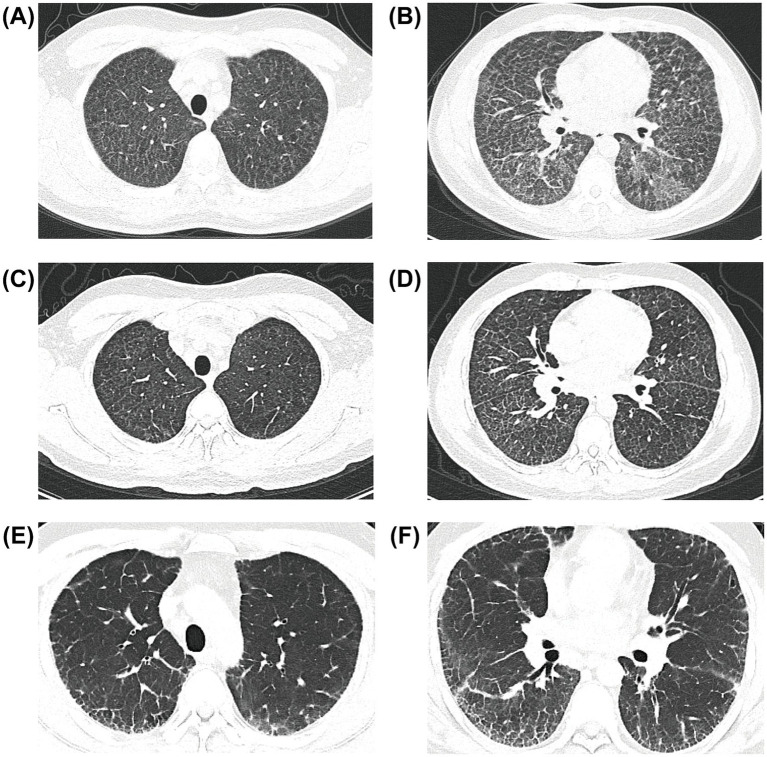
High-resolution computed tomography (HRCT) scans showing ground-glass opacification and interlobular septal thickening. The patients were scanned upon hospitalization in February 2025 (A,B). HRCT revealed reduced ground-glass opacification 40 days after whole-lung lavage (C,D). HRCT at 10 months after whole-lung lavage revealed a continuous reduction in ground-glass opacification; however, the reticular pattern increased (E,F).
